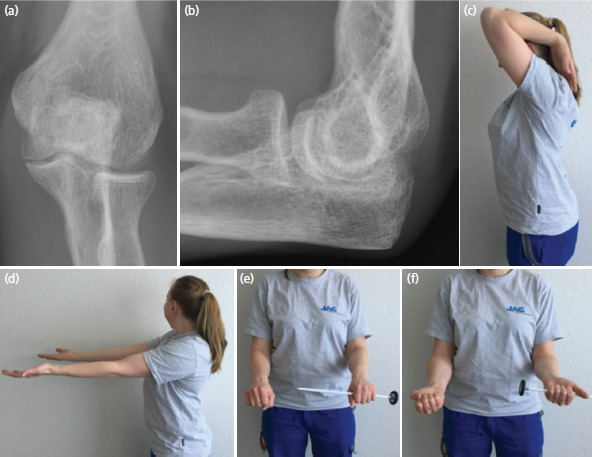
Radiographic and clinical result 10 years after tumour resection and autologous bone grafting. (a) Anteroposterior and (b) lateral radiographs of the elbow show a normal joint without tumour recurrence and without joint space narrowing. Photographs show normal (c) flexion, (d) extension, (e) pronation and (f) supination.
